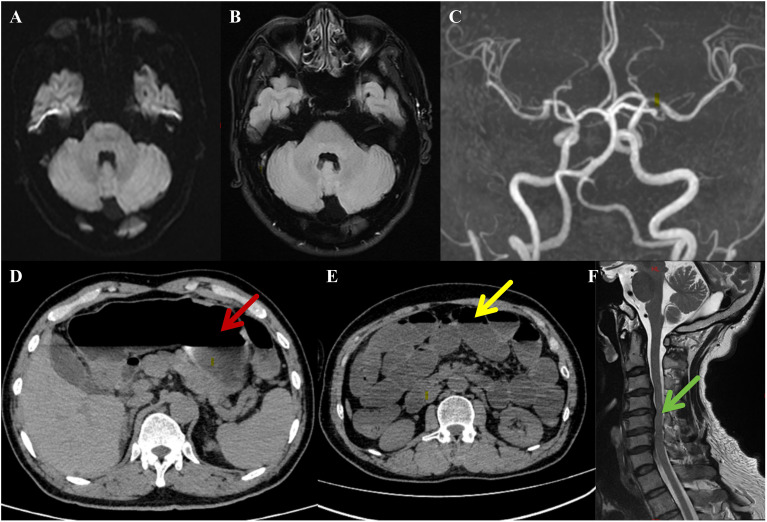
Imaging findings of the patient. Brain magnetic resonance imaging (MRI) showed normalities (A–C). Whole abdominal Computed Tomography (D, E): distension of the gastric lumen (red arrow) and small intestinal loops (yellow arrow), with gas and fluid accumulation, and multiple air-fluid levels observed. Cervical spine T2-weighted MRI (F): C3–7 intervertebral disc bulge and protrusion with C4–6 spinal canal stenosis (green arrow).
